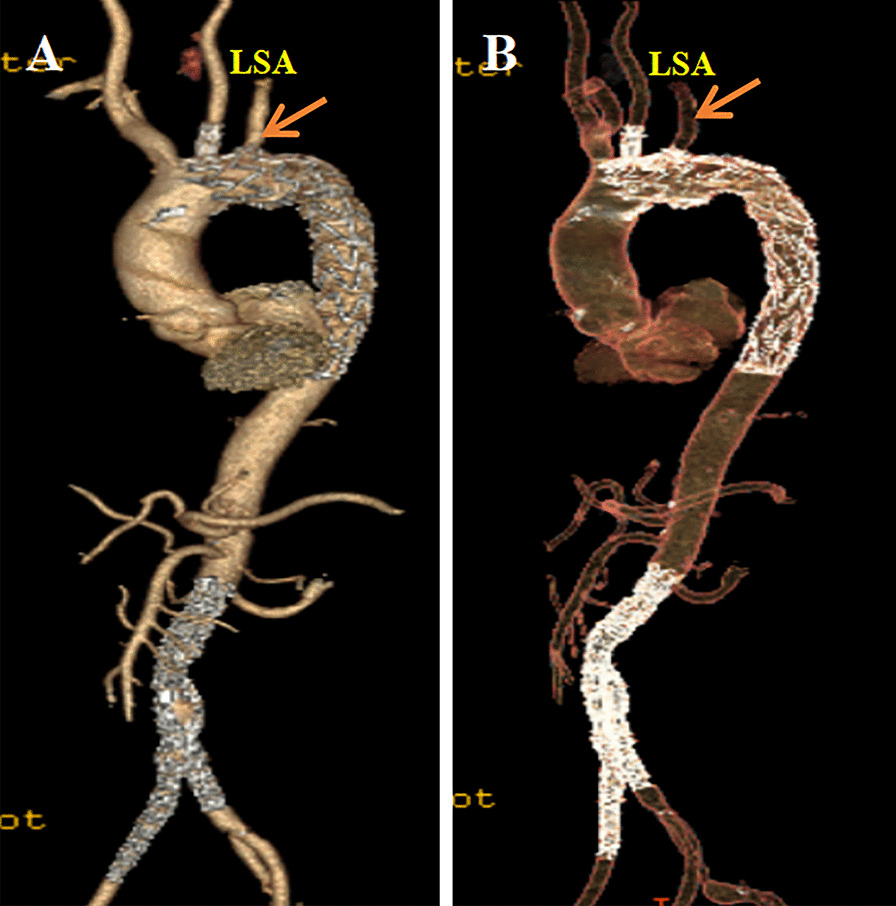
A A case we gave up implanting covered short self-expanding stent graft to LSA due to unsatisfactory dilation effect of in-vitro fenestration. B Postoperative CTA comfortingly show the perfect patency of LSA through the aperture of the in-vitro fenestration and there was no membrane leakage
